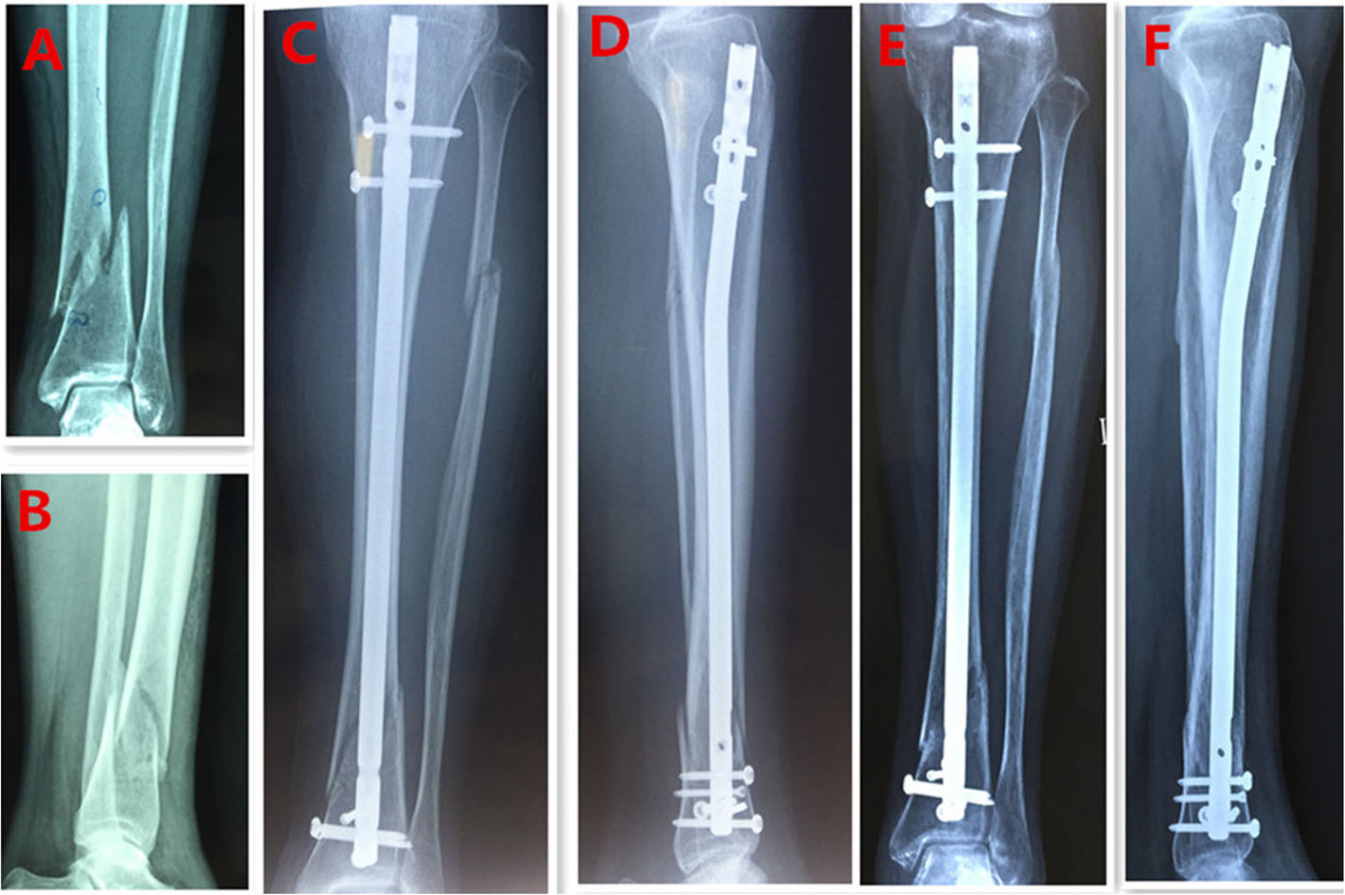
Radiographs of a case of union after closed reduction using the infrapatellar approach were presented. a, b Preoperative anteroposterior (AP) and lateral views. c, d AP and lateral views postoperatively. e, f AP and lateral views 5 months postoperatively
